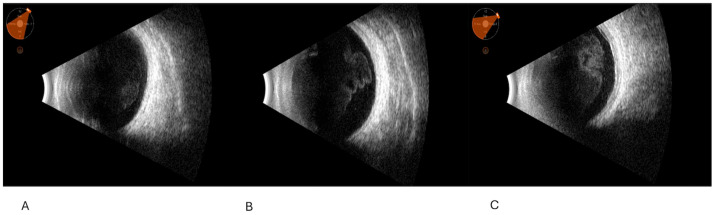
Ultrasound findings in mild, moderate, and severe vitritis. (A) Slight vitreous corpuscles collected in “clouds” with minimal increase in chorioretinal thickness (syphilis); (B) moderate vitreous corpuscles with a high mobility, organized in posterior clusters. The main aspect of the vitreous body is its high cellularity, which is accompanied by abnormalities of the posterior profile (tuberculosis); (C) abundant vitreous corpuscles with structured free hyperreflective membranes in the vitreous, associated with multiple posterior profile abnormalities, including posterior membranes with subretinal fluid, and thickening in the macular region (birdshot chorioretinopathy with choroidal neovascularization, CNV).
